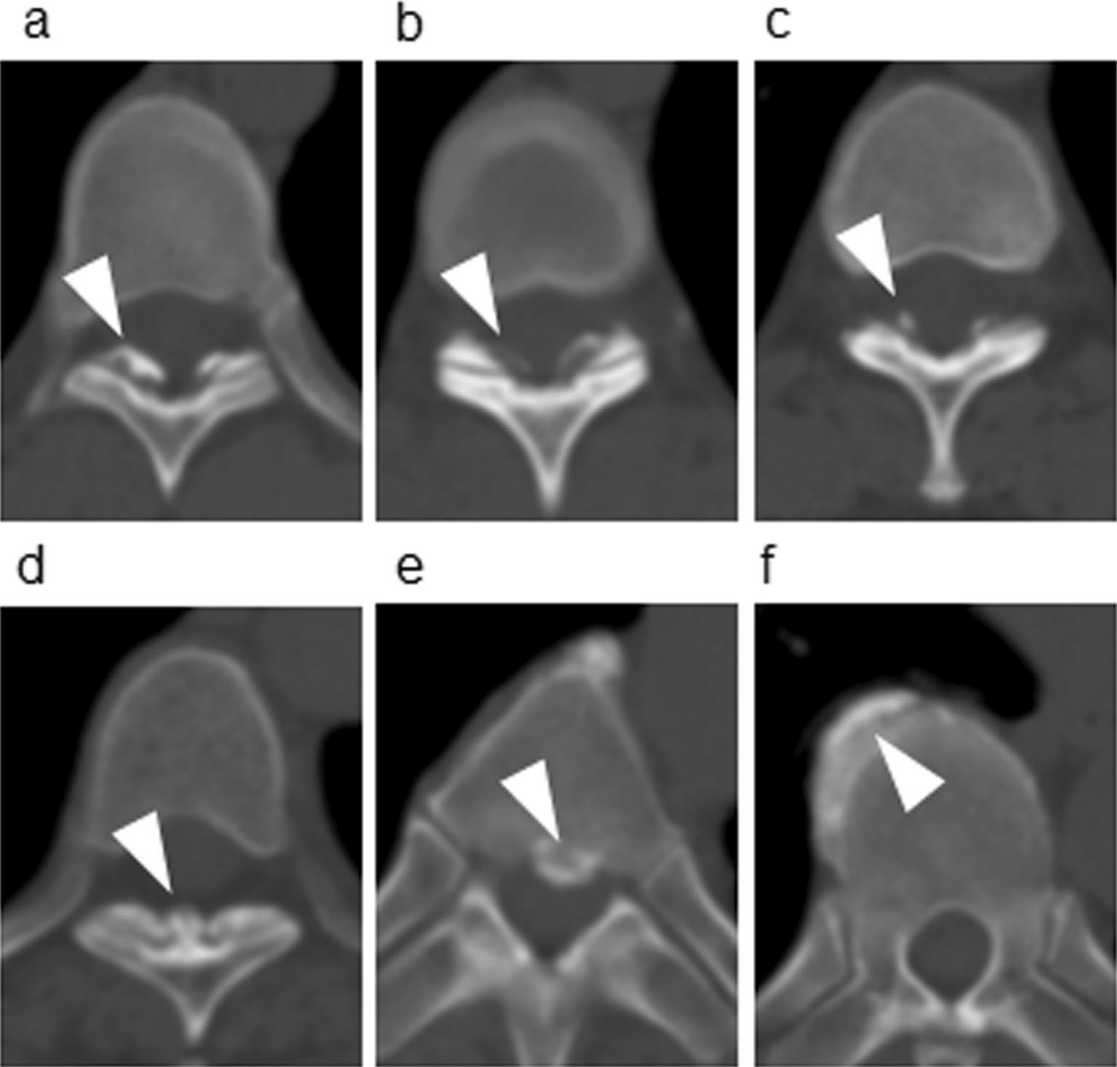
Representative examples of OLF, OPLL, and OALL (indicated using arrowheads) on computed tomography images. (a) OLF with thickness ≥ 2 mm. (b) OLF with thickness ≥ 1 mm. (c) OLF clearly visible at 1 mm thickness. (d) A mushroom-shaped OLF localized at the center of the laminae. (e) OPLL with thickness ≥ 2 mm. (f) OALL located in the paramedian region of the anterior vertebral body with a thickness ≥ 4 mm. *OLF* ossification of the ligamentum flavum, *OPLL* ossification of the posterior longitudinal ligament, *OALL* ossification of the anterior longitudinal ligament.
